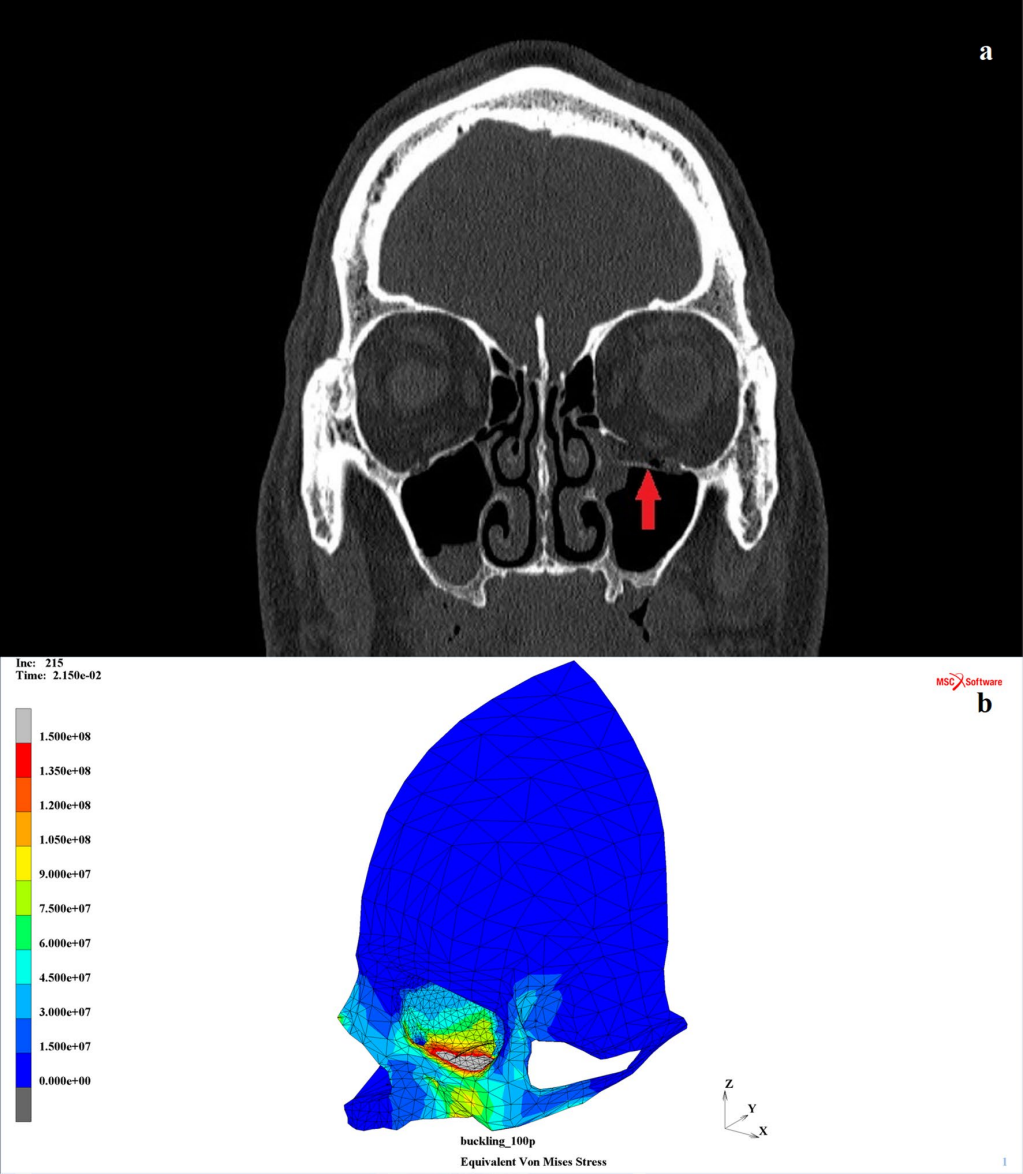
CT scan analysis and the impacting load assessment: (a) exemplary patient (male, 48) with substantial-scale fracture of the lower wall of the left orbit (red arrow) and (b) corresponding scale of the orbital damage for the time t = 21.5 ms in the MOBOSE^30^.
